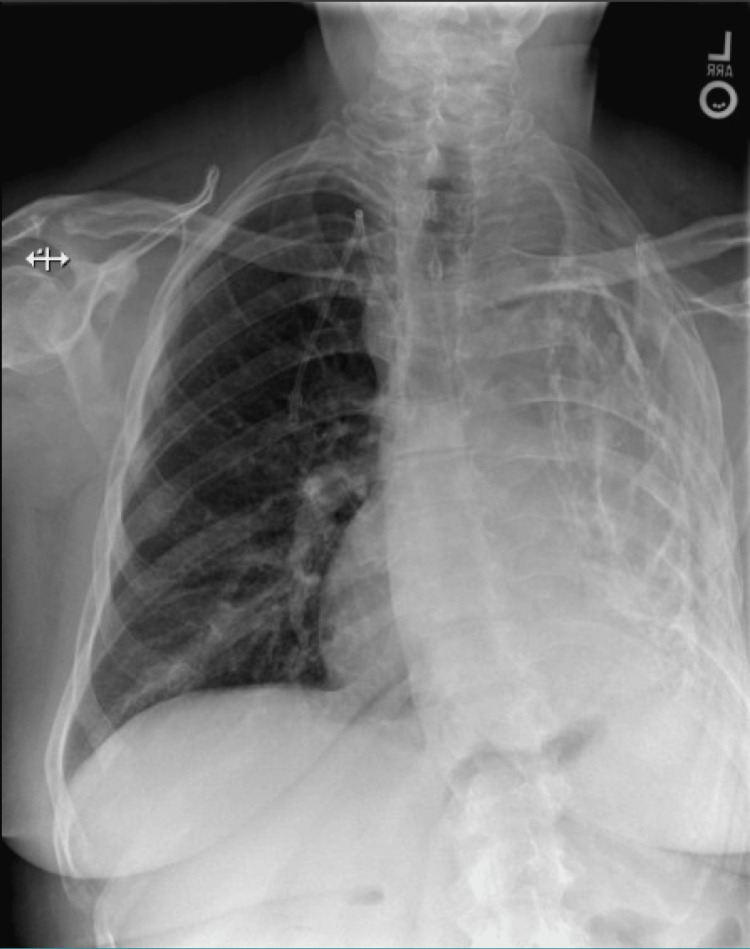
Chest radiograph depicting changes post left pneumonectomy (January 2022).
